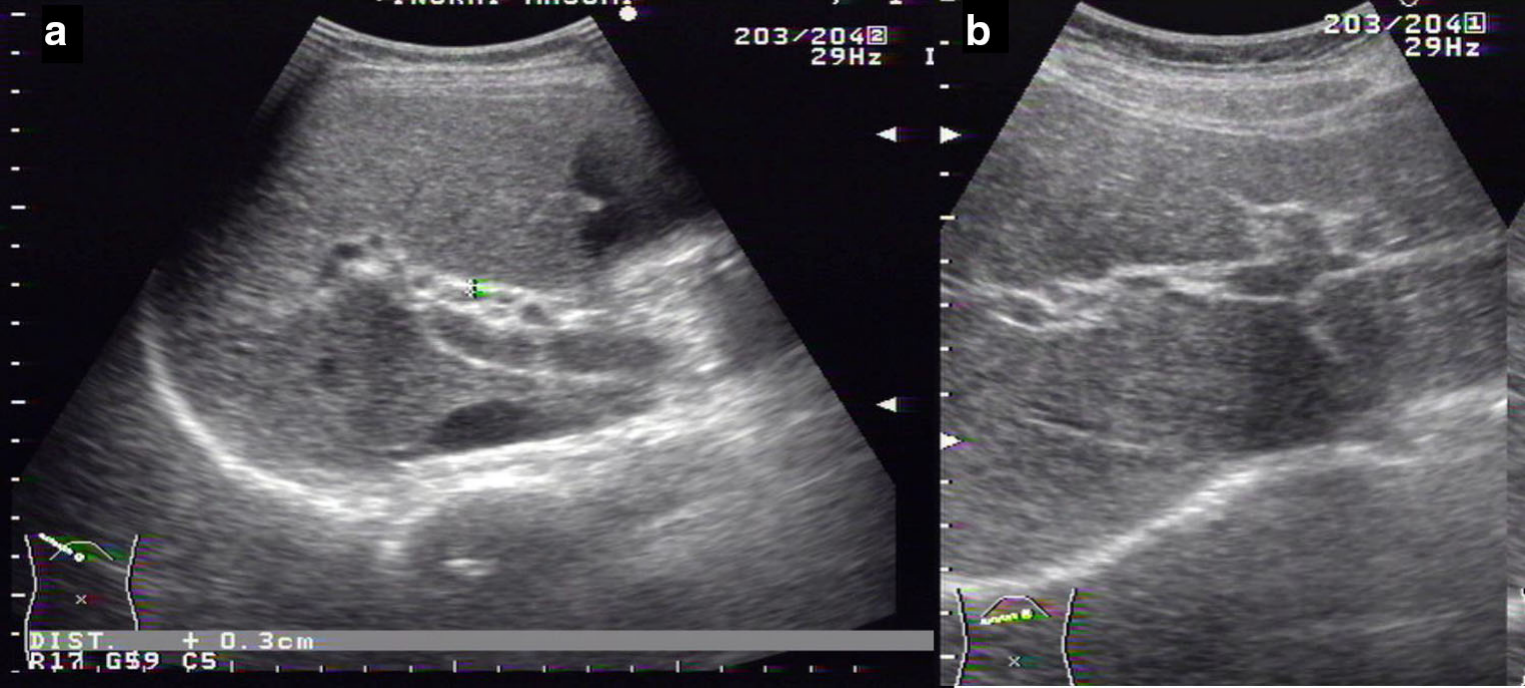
Ultrasound Image of PSC. a Common bile duct wall thickening is observed. (right intercostal scanning). b Intrahepatic bile duct at the umbilical region are dilated and have thickened irregularly. (wide probe scanning)
